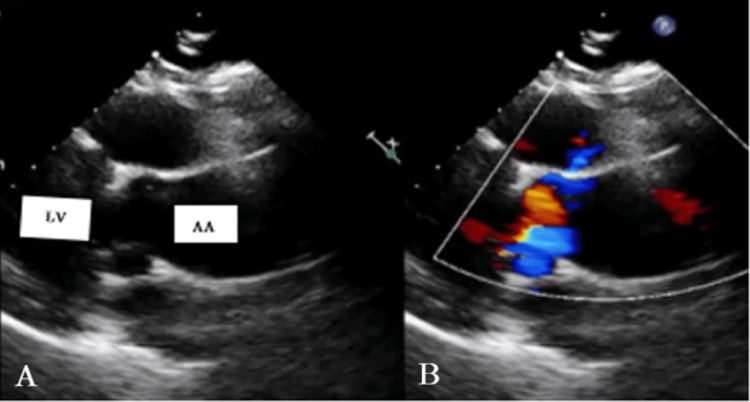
(A) Parasternal long-axis view showing the ascending aorta and the left ventricle. (B) Parasternal long-axis view with color. AA = ascending aorta; LV = left ventricle
